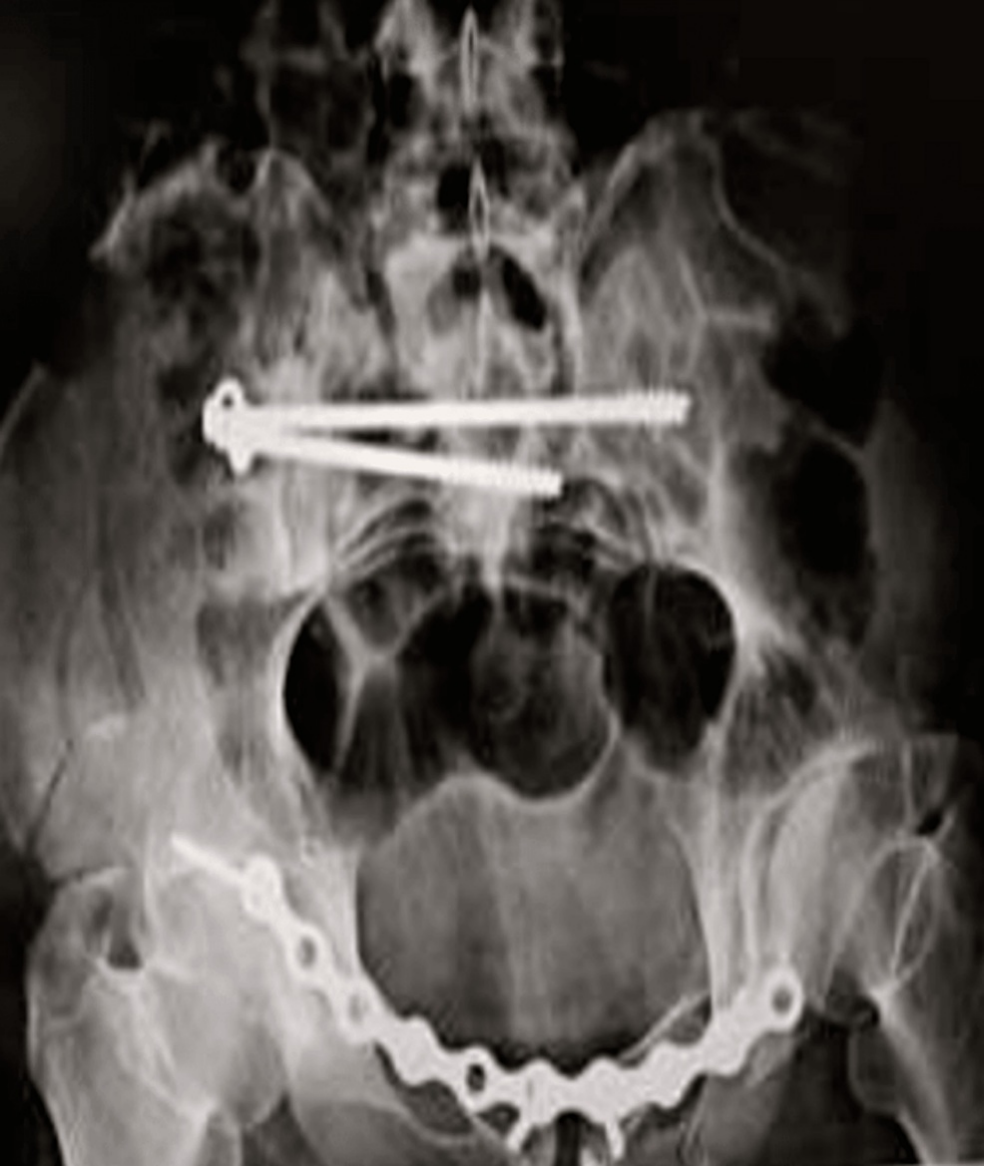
Post-operative X-ray showing pelvic inlet
